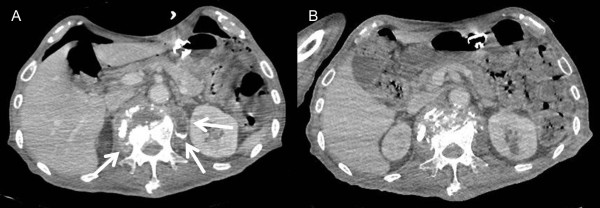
Computed tomography (CT) findings of the multifocal bone and lymph node tuberculosis with concomitant psoas abscess. Contrast CT images of a comminuted fracture of the L1 vertebral body and abscesses in the bilateral psoas major muscles (arrows) performed (A) before treatment and (B) after percutaneous abscess drainage and 6 months of antitubercular drug treatment.
